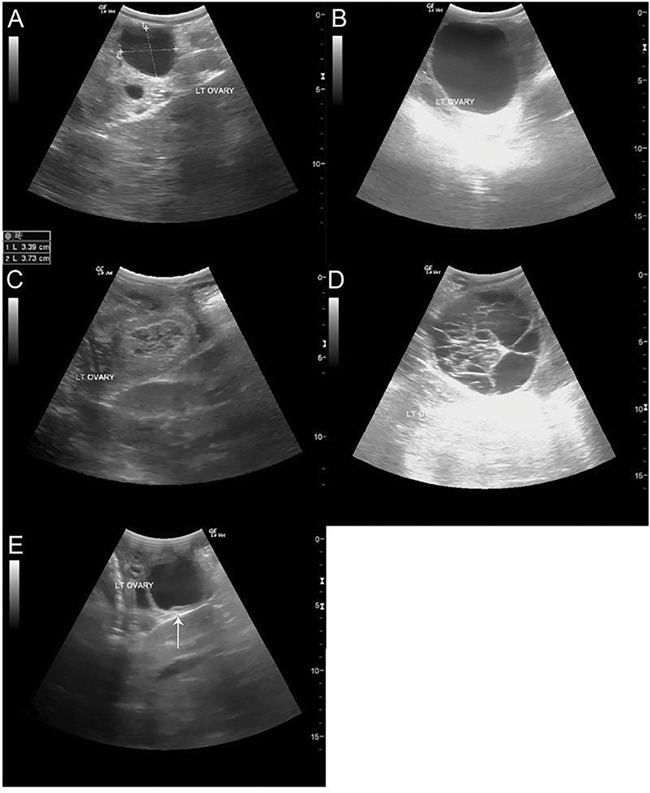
Ultrasound images of follicle types observed during study period. Representative images of follicle types observed during study period. (A) Dominant follicle that was treated with GnRH and resulted in ovulation. (B) Dominant follicle that was not treated and grew to ~60 mm, then regressed without ovulating. (C) CL following GnRH treatment. (D) HAF that formed following GnRH treatment with failure to ovulate. (E) Unstimulated follicle displaying a visual indicator of atresia, arrow indicates the lifting granulosa cell layer, which subsequently regressed without ovulating.
